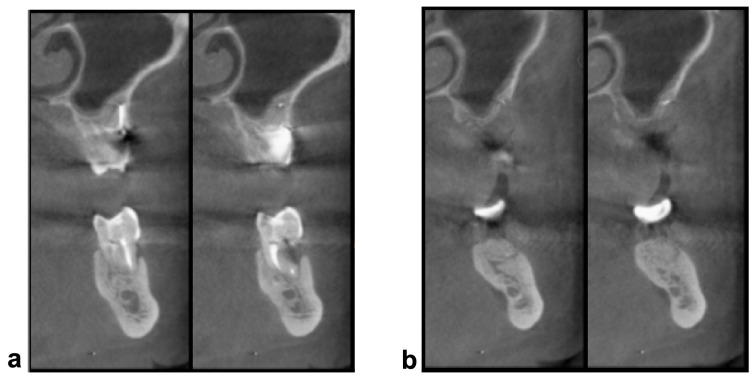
(a) CBCT scans acquired before grafting procedures; (b) CBCT scans acquired after 4 months.
